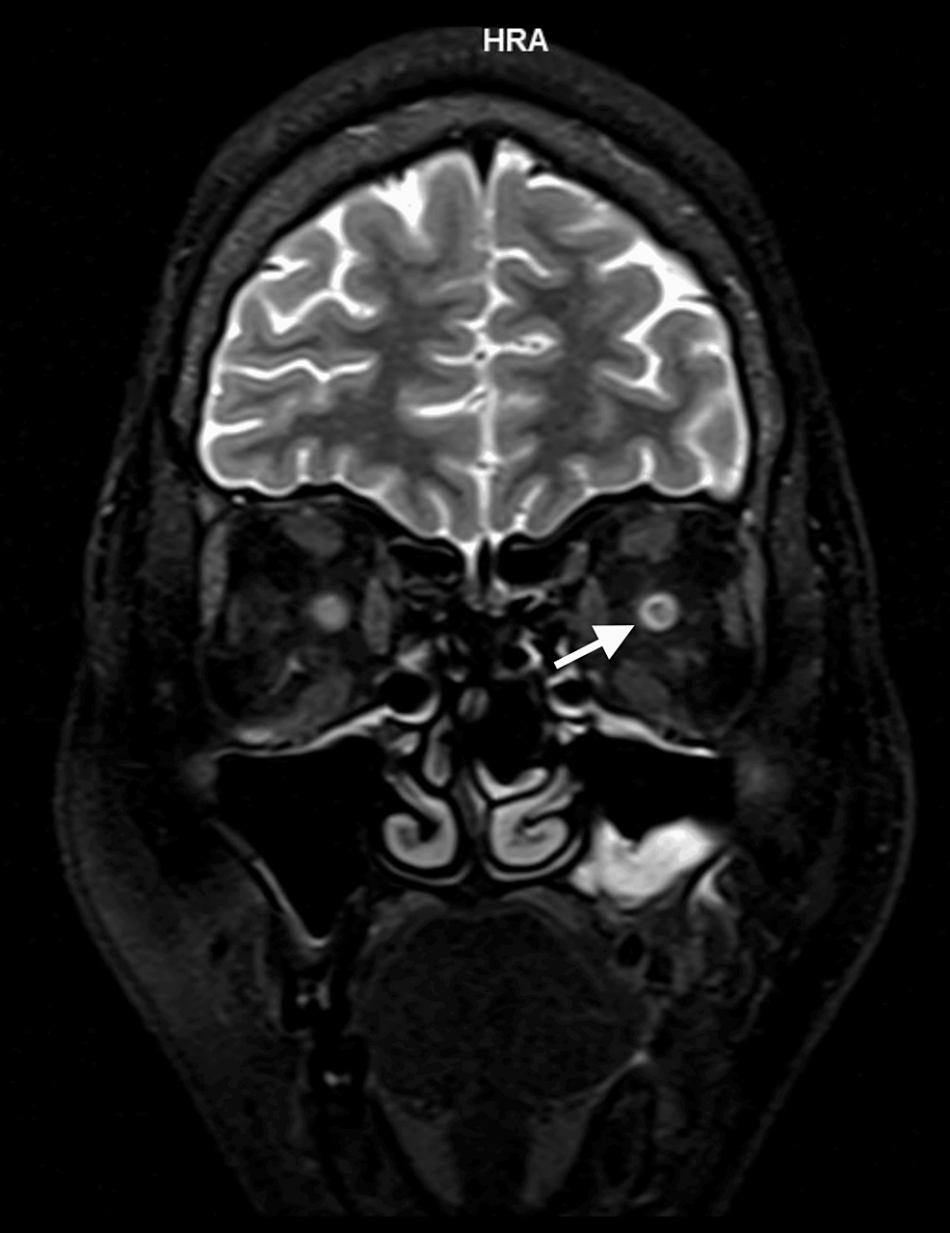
MRI film coronal view: left perineural enhancement ("doughnut sign") The white arrow shows the left eye optic nerve sheath enhancement.
